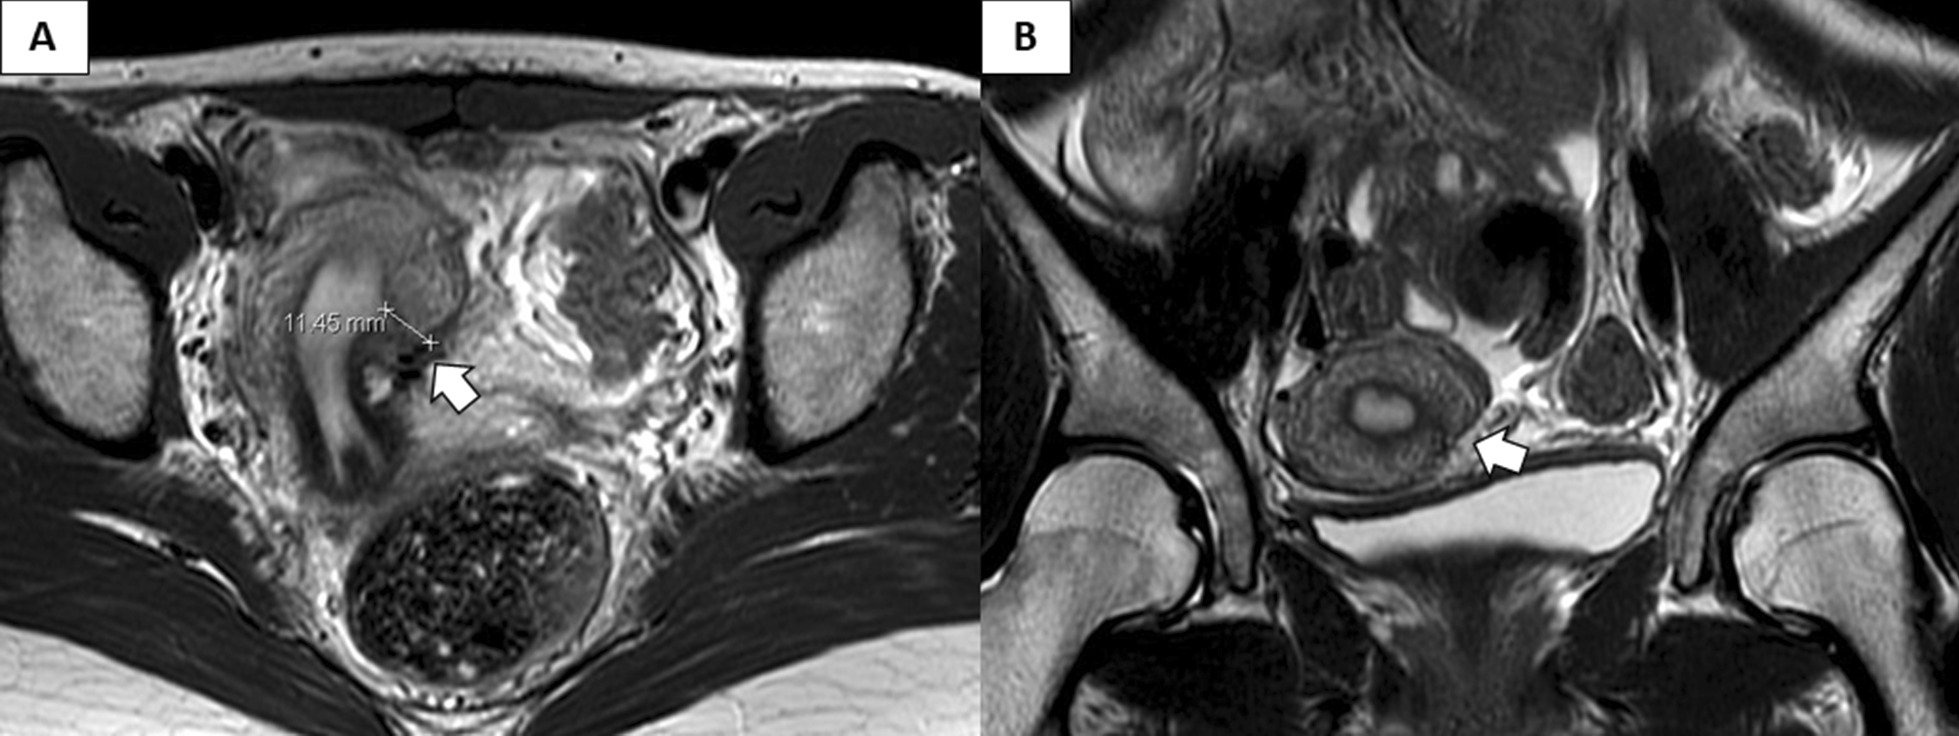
The findings of a follow-up MRI scan 3 years after the surgery. A uterus with a thick muscular layer (white arrow) was observed via the axial (a) and coronal (b) planes, and presented with a normal uterine appearance
